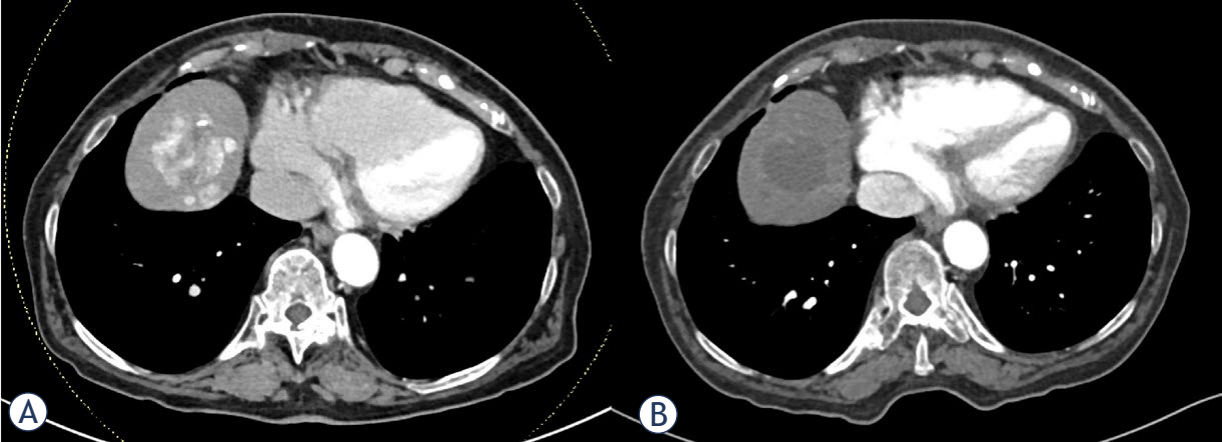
CT slices showing a mRECIST partial response to idarubicin-loaded drug-eluting microspheres (DEMIDA) - transarterial chemoembolization (TACE) in the VIII. segment of cirrhotic liver. (A) Baseline CT showing multifocal hepatocellular carcinoma (HCC). The diameter of the largest tumour was 38 mm; (B) Only minimally enhancing largest target lesion at the first treatment response evaluation, the diameter of viable lesion was 4 mm.
